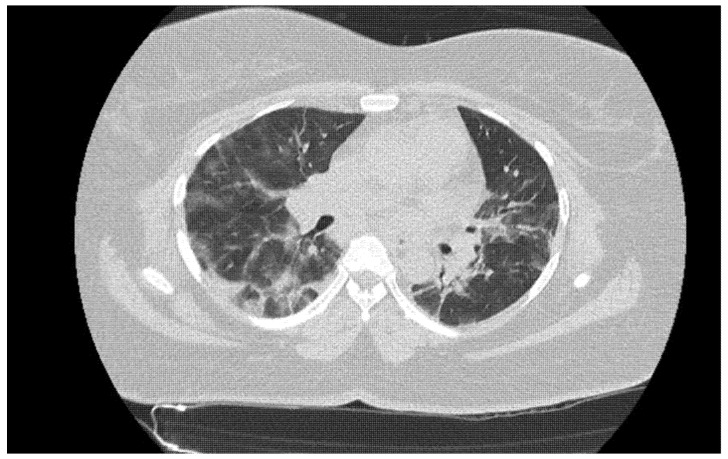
Unenhanced chest CT in a 44-year old-woman with dyspnea and fever for the previous 3 days. Bilateral ground-glass opacities are present, with subpleural predominance and associated interstitial septal thickening. Findings were consistent with typical COVID-19 pneumonia, encompassing 25 to 50% of the total lung parenchyma.
